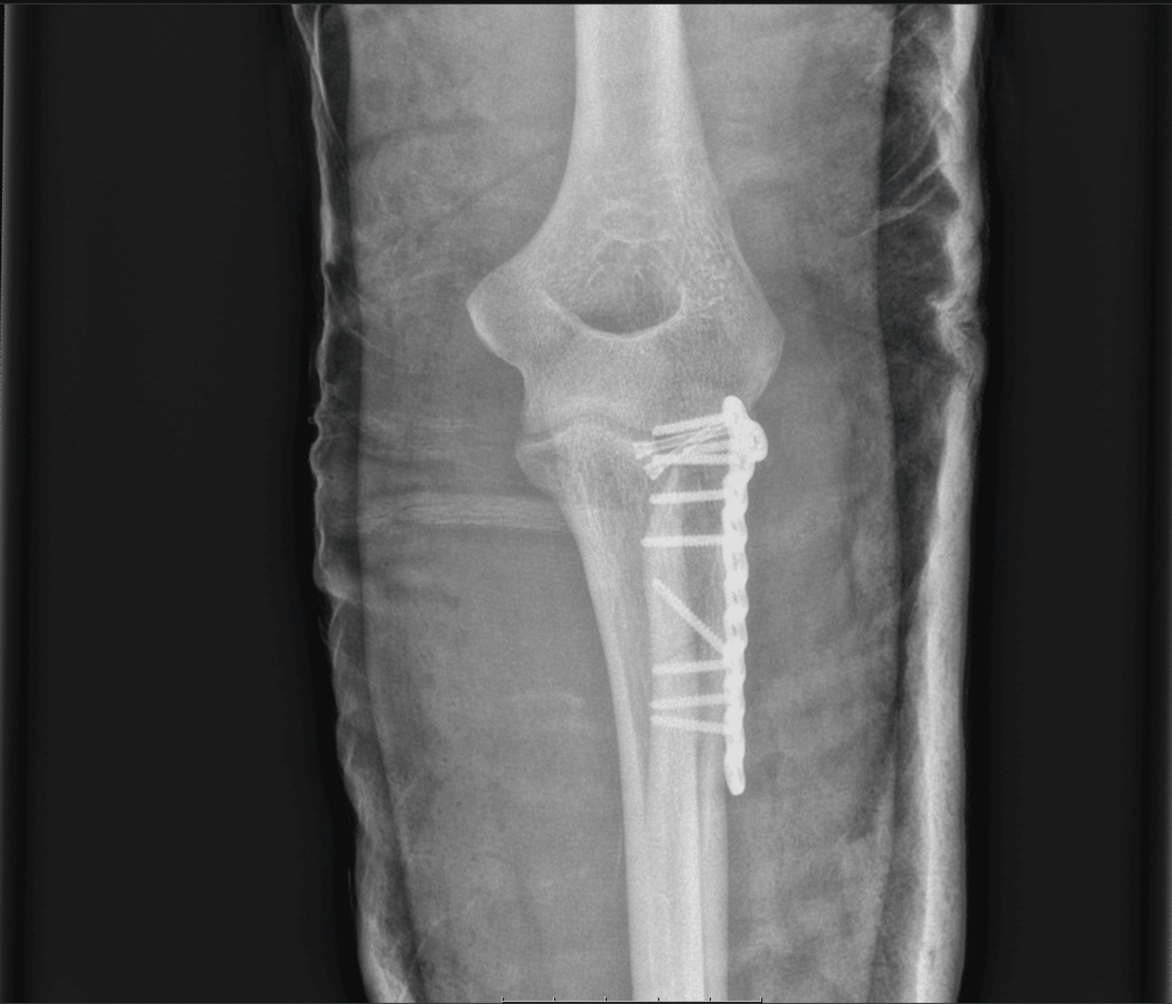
Anteroposterior view of postoperative radiograph at two weeks
